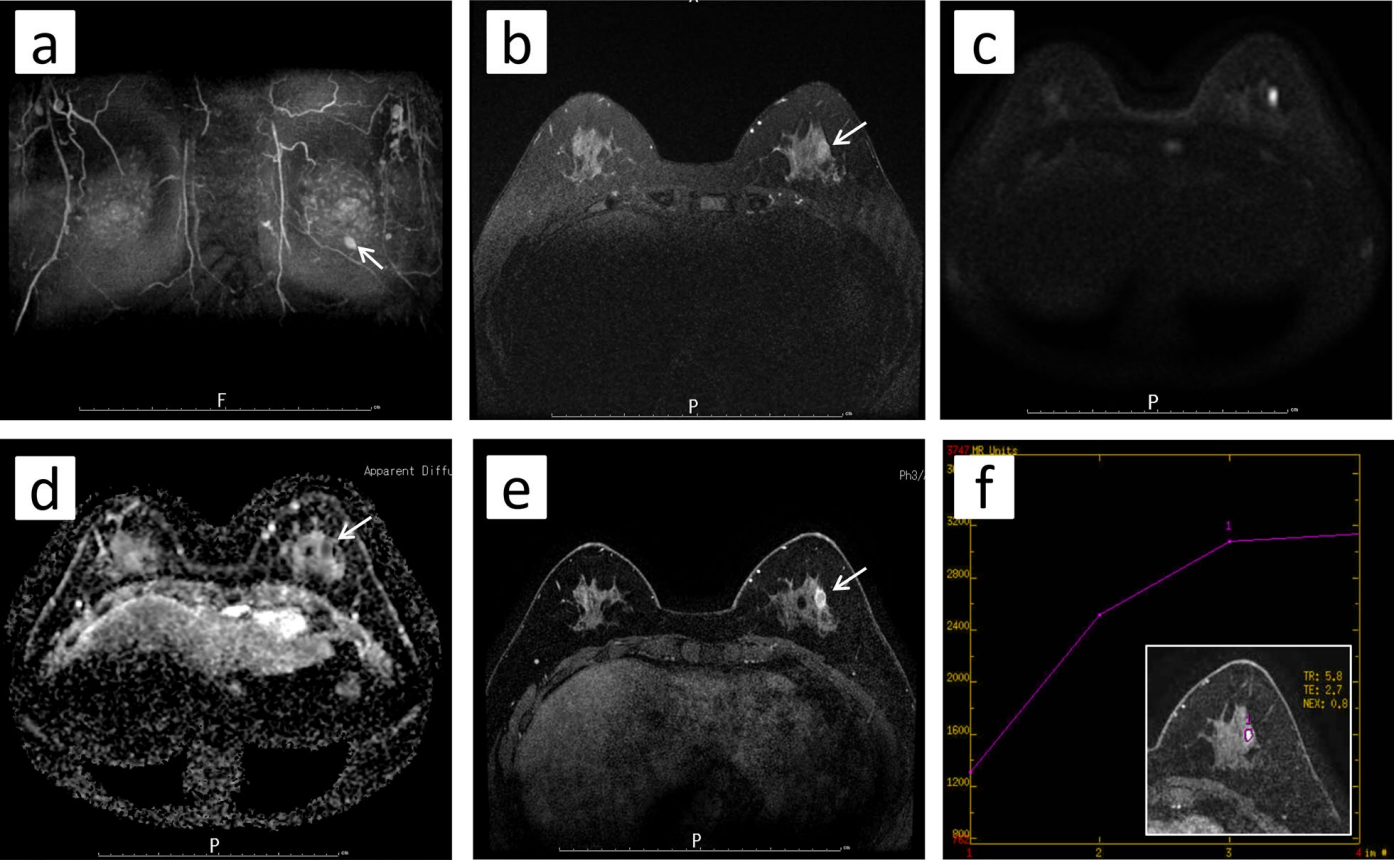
Magnetic resonance imaging. a A 1.2-cm-diameter mass at the lower outer portion of the left breast. b T2-weighted imaging: Mildly high signal intensity at the periphery and center of the tumor. c Diffusion-weighted images: high signal intensity. d Apparent diffusion coefficient map: low signal intensity. e Dynamic contrast-enhanced magnetic resonance imaging: hyperintensity in the early phase. f Time–intensity curve: intermediate enhancement in the initial period and a plateau in the delayed period
